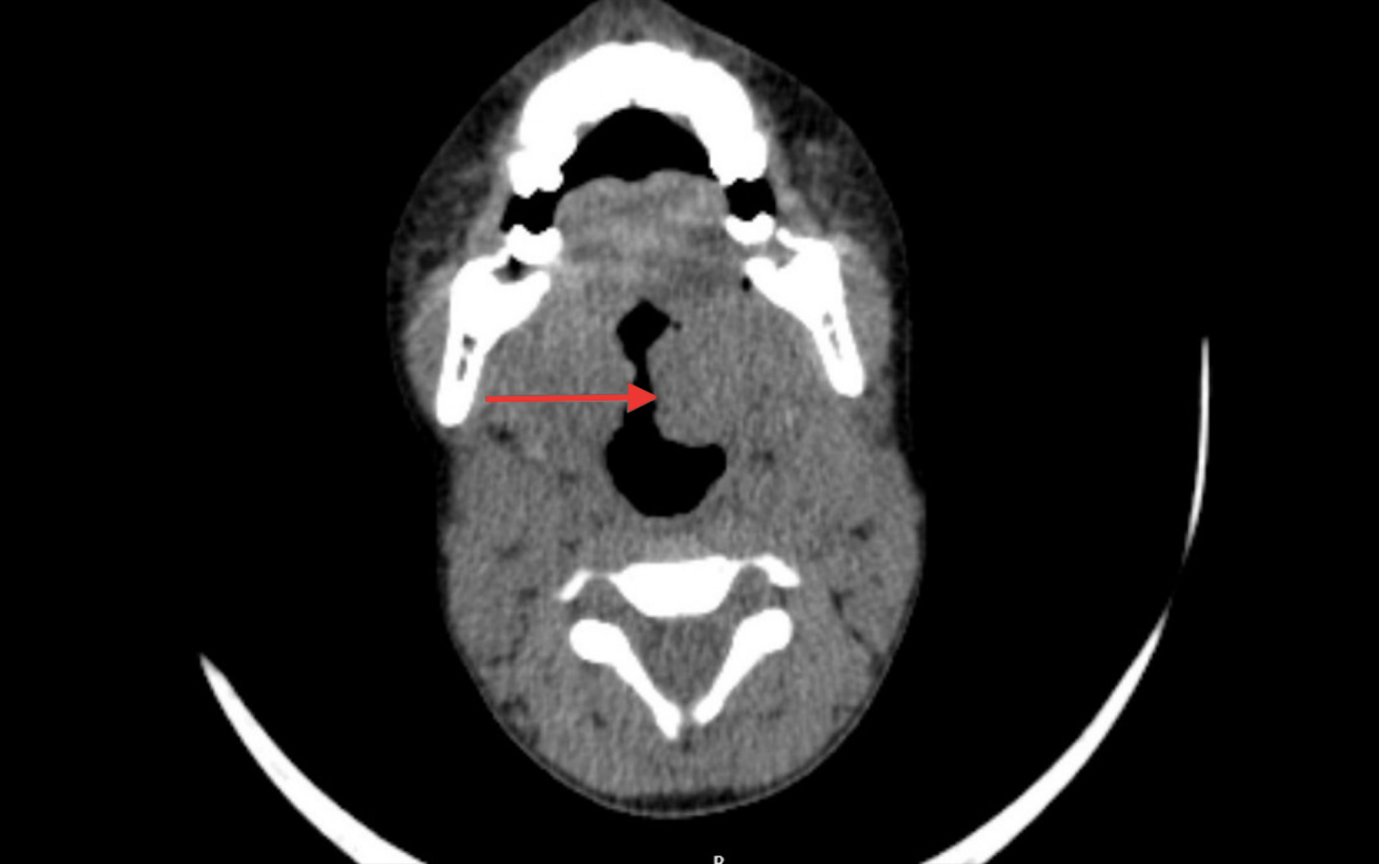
Axial cut of CT showing considerable enlargement of the tonsils (Red arrow).
